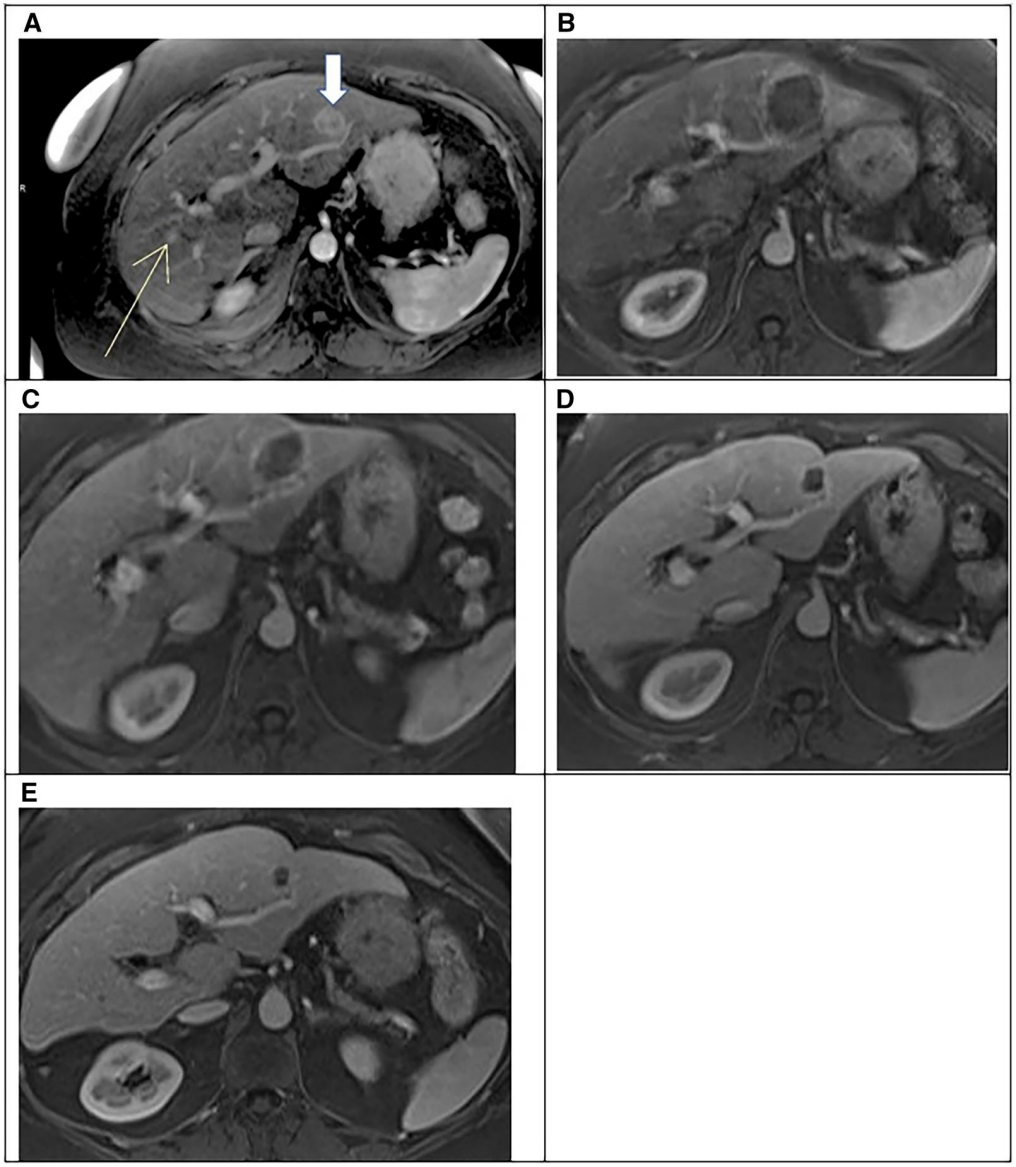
Images from patient of #HOPE4LIVER study,^11^^,^^13^^,^^49^ showing (A) a 2-cm hepatocellular carcinoma in segment II (wide arrow), which was treated with histotripsy and a 1.5-cm hepatocellular carcinoma in segment VIII (thin narrow), which was treated with image-guided microwave ablation. Follow-up MRI at (B) 1-day post-histotripsy showing a non-enhancing treatment zone. Subsequent follow-up at (C) 1 month, (D) 6 months, and (E) 1 year showing progressive involution of the treatment zone.
